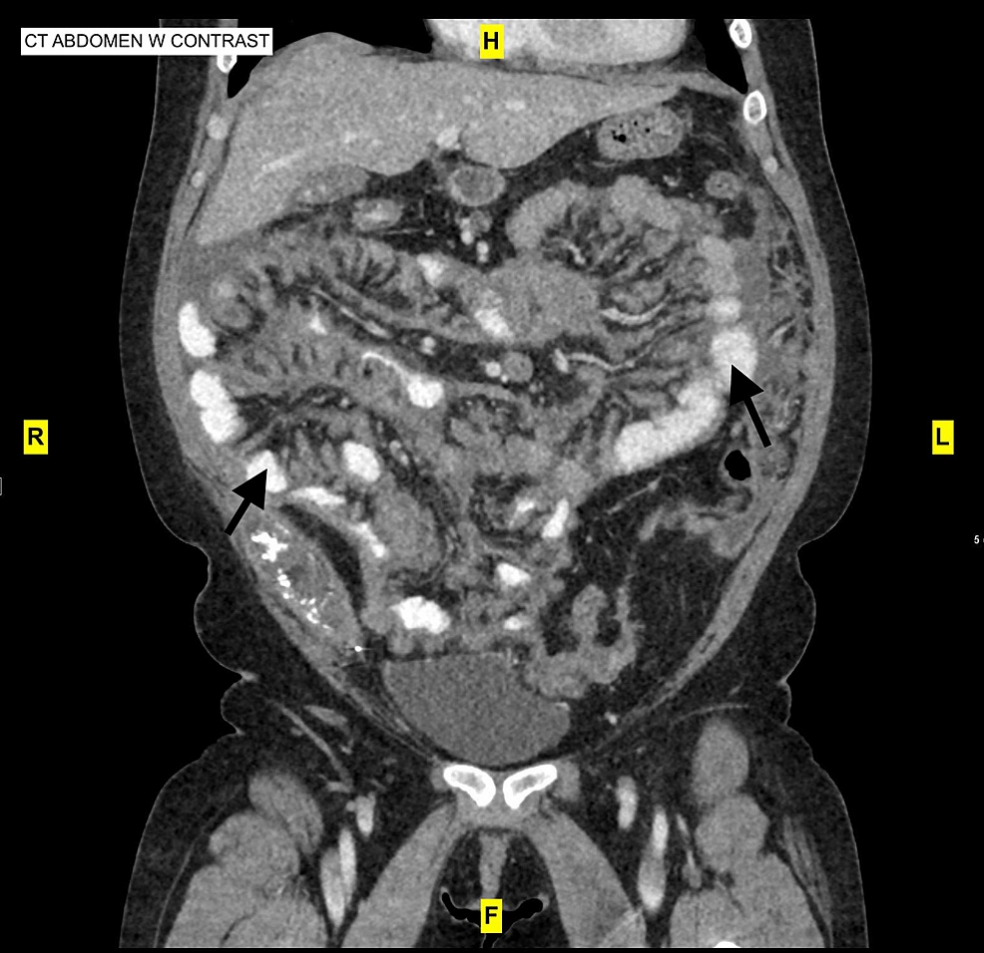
CT abdomen with contrast showing peritoneal nodularity, stranding, and omental thickening diffusely throughout the abdomen with small perihepatic ascites
